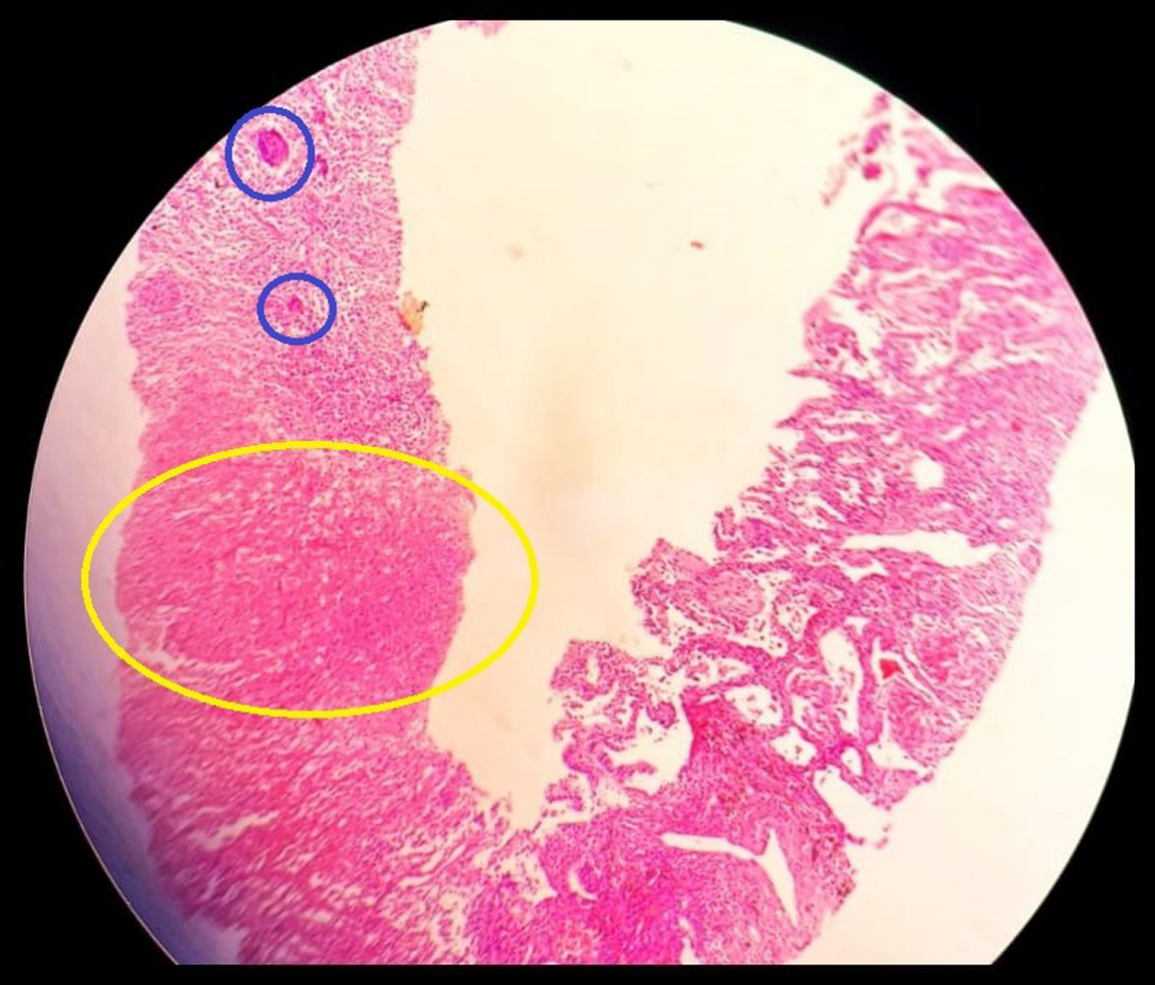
CT‐guided lung biopsy revealing the presence of chronic granulomatous inflammation characterized by Langerhans‐type multinucleated giant cells (blue circle) and widespread areas of caseous necrosis (yellow circle).
